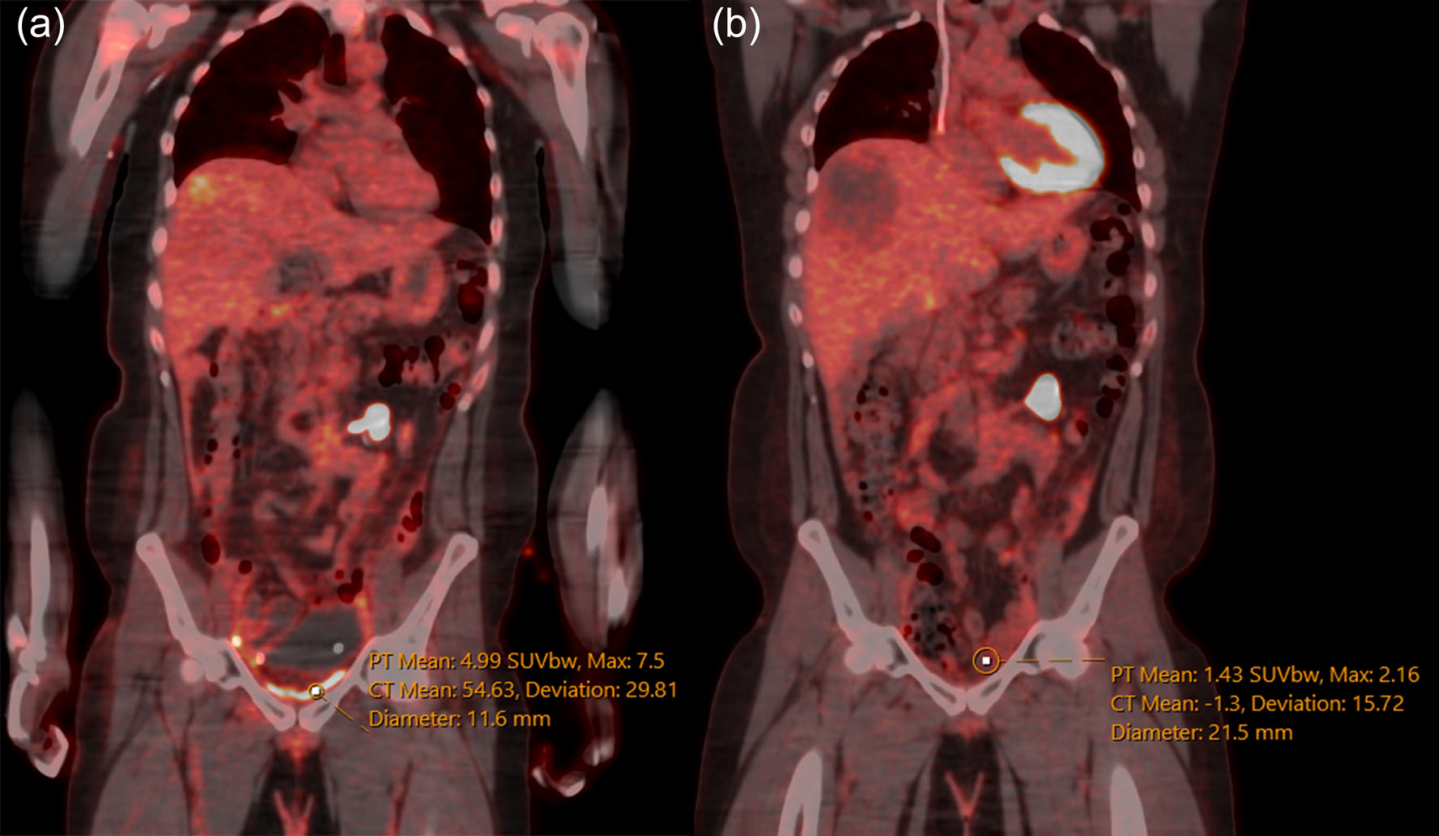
(a) PET/CT fusion coronal view at 1 month postoperatively. (b) PET/CT fusion coronal view at 9 months postoperatively.
